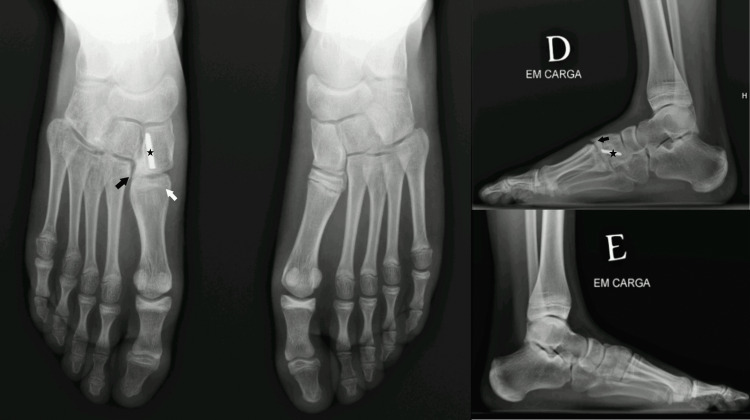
Weight-bearing bilateral X-rays: 18 months post-operative. The black arrow represents the spur on the medial cuneiform; the white arrow represents the premature physeal arrest on the base of the first metatarsal; Star represents the part of the screw-retained in the medial cuneiform.
